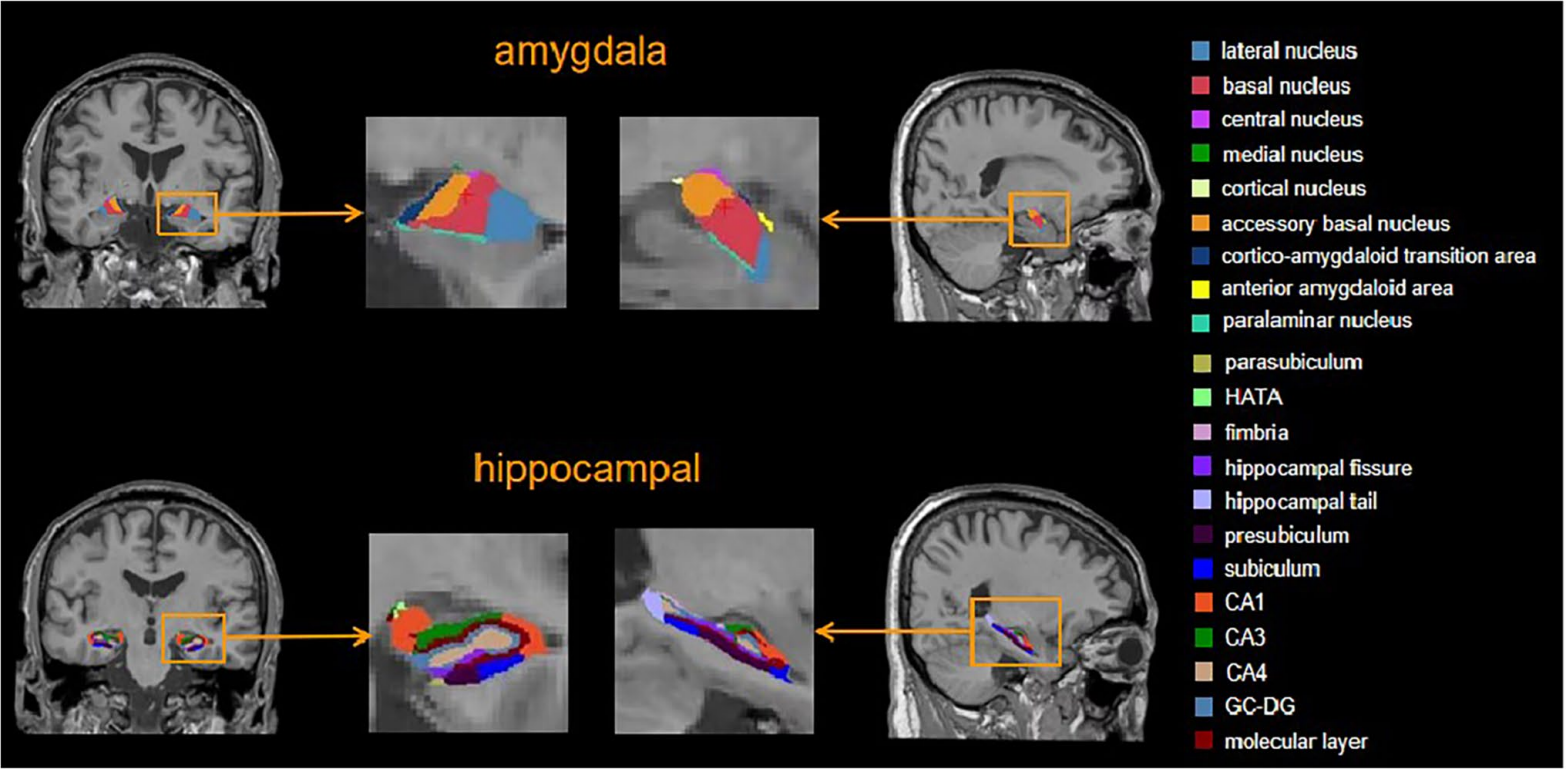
Coronal and sagittal 3D-T1-weighted images from the automated segmentation of Freesurfer hippocampal and amygdala subregions segmentation from a healthy individual. Abbreviations: GC-DG, Granule cell layer of the dentate gyrus; HATA, Hippocampus-amygdala transition area
